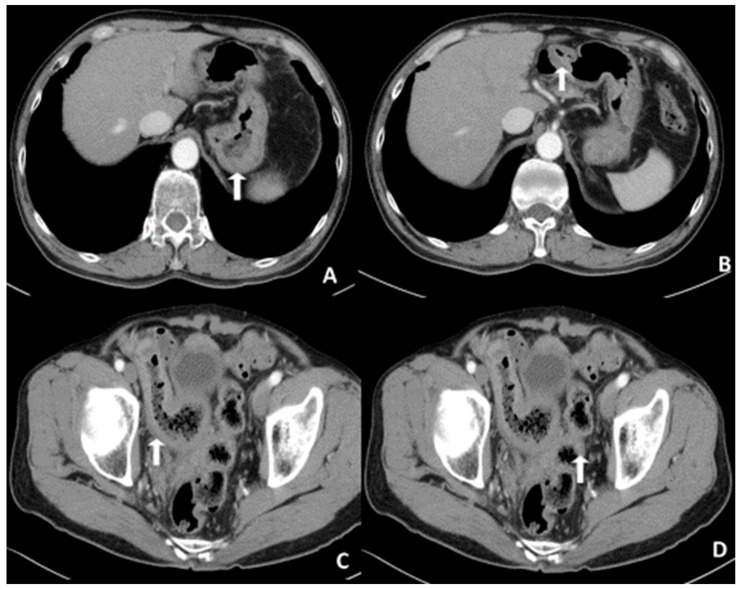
The abdominal CT scan revealed MALToma in the stomach (A), duodenum (B), terminal ileum (C), and sigmoid (D).
